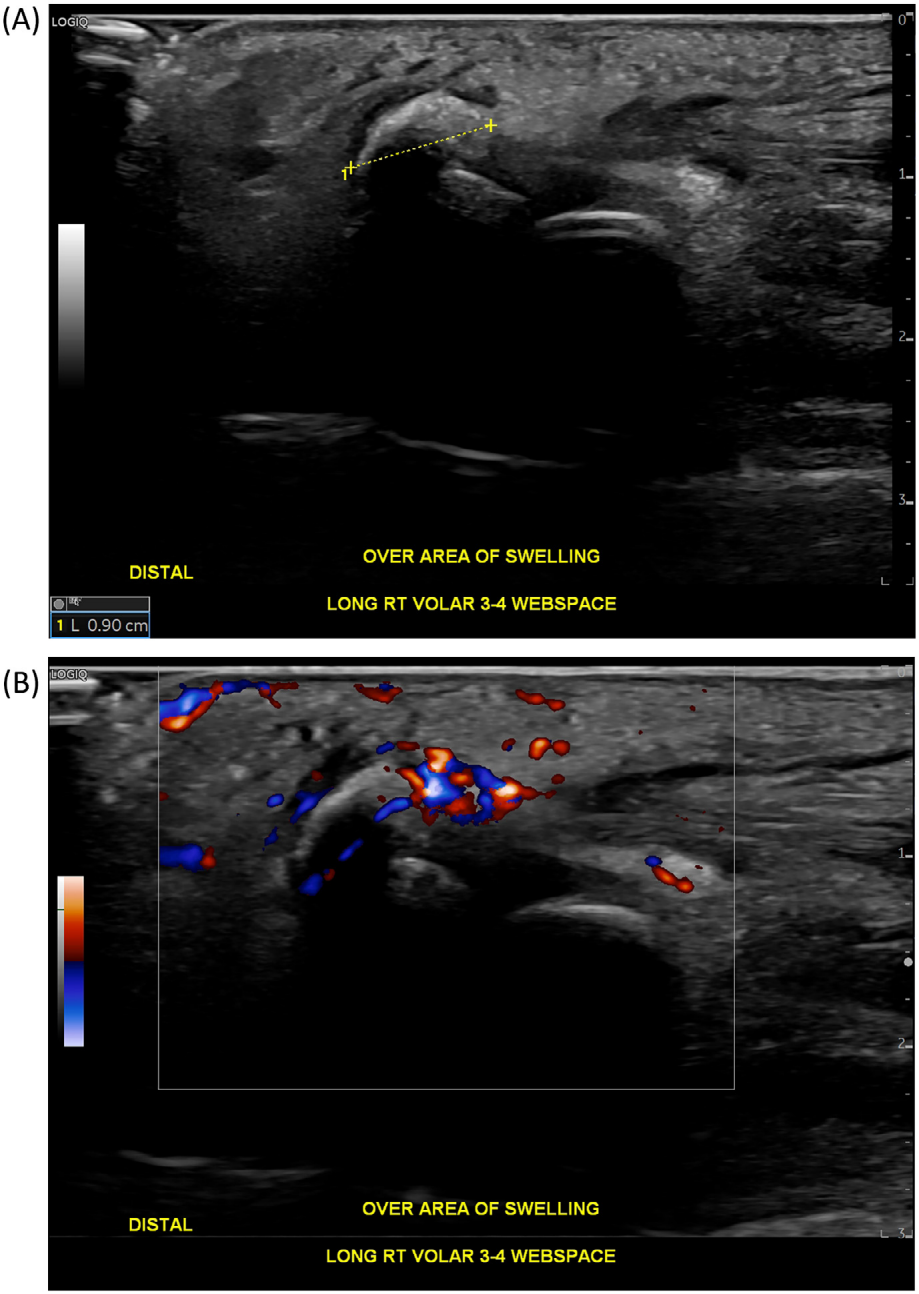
(A) Longitudinal ultrasound image of the right hand demonstrates globular calcification with acoustic shadowing at the third webspace at the level of the MCP joint. (B) Corresponding color Doppler ultrasound image shows hyperemia around the calcification, indicative of inflammation.
